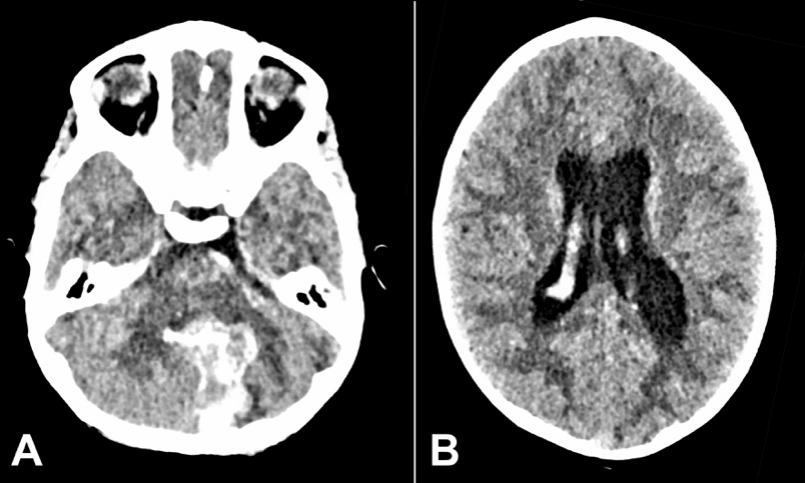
A – Brain CT on the first presentation. Intracerebral hemorrhage in the cerebellar vermis and medial aspect of the left cerebellar hemisphere with intraventricular extension; B – Brain CT on first presentation showing supra-tentorial hydrocephalus.
